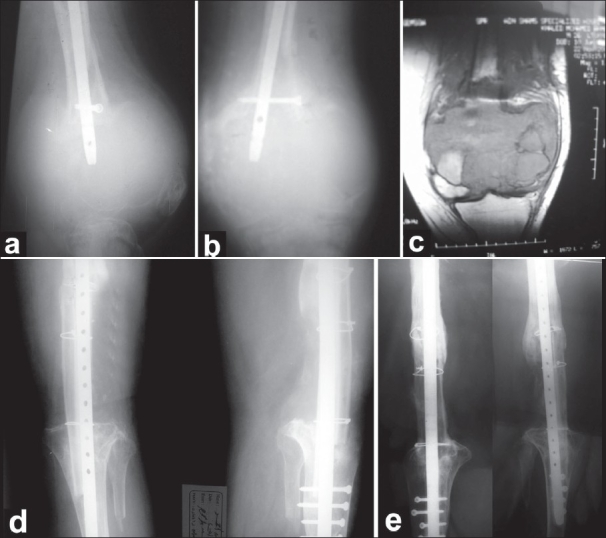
Case 2. (a and b) Preoperative X-rays showing extensive bone destruction at the distal femur with the intramedullary nail inside. (c) MRI showing extensive soft tissue involvement of all compartments. (d) Postoperative X-rays. (e) Follow-up X-rays after 48 months showing graft union and hypertrophy.
